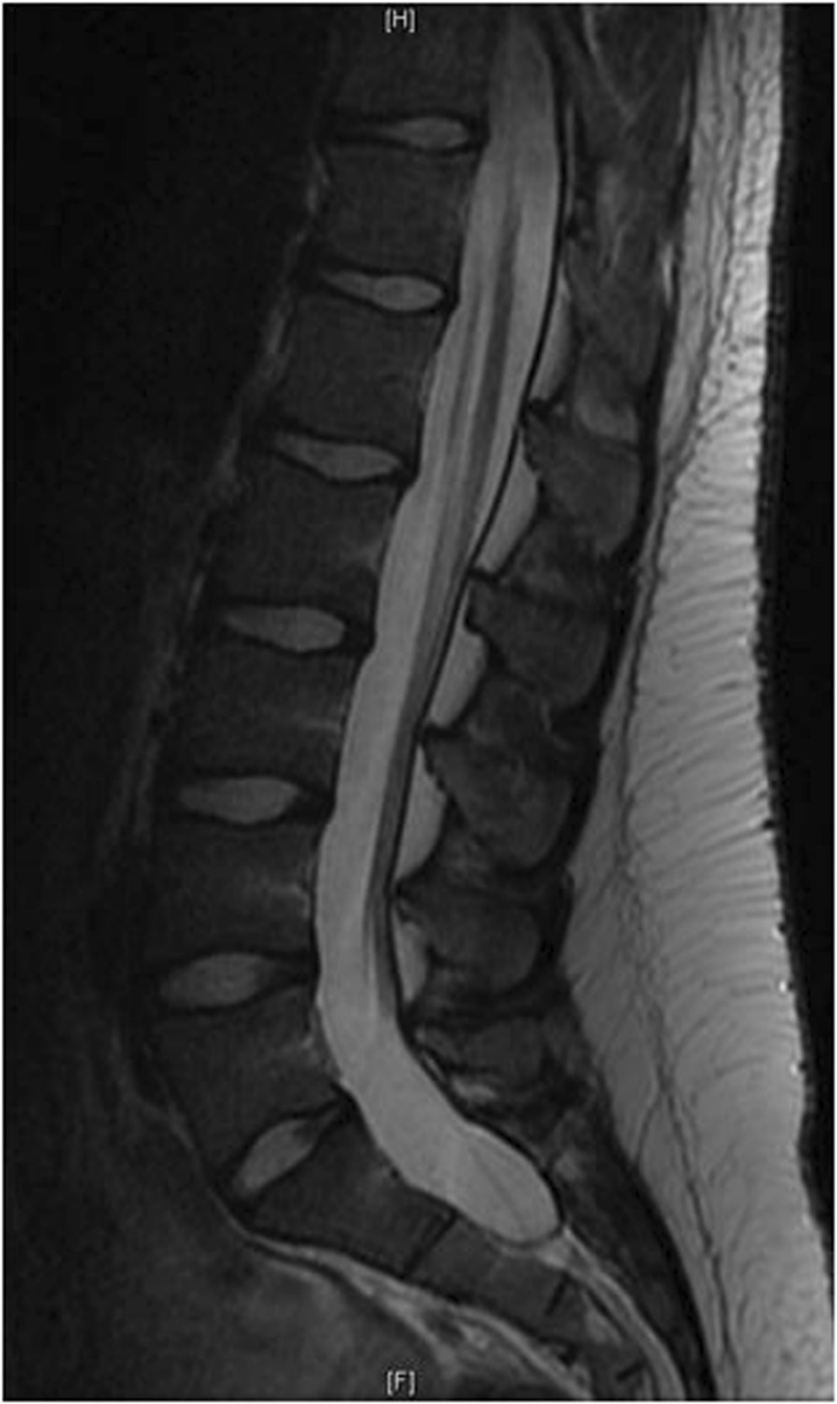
Pre-operative MRI of a representative patient with Marfan syndrome.
